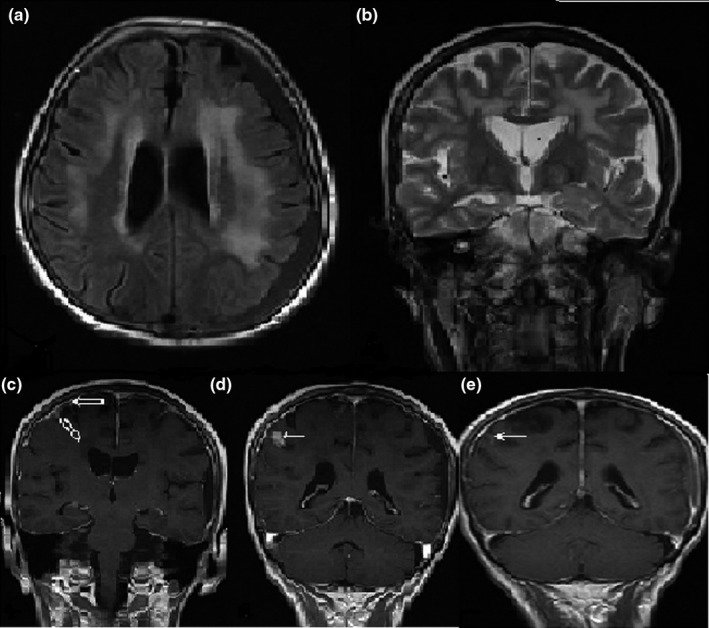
Axial FLAIR (a) and coronal T2‐weighted (b) images of patient with sensorineural hearing loss showed diffuse hyperintense signal changes bilaterally in the subcortical and periventricular deep white matter and the subdural hygroma on the left. In postcontrast coronal (c) series, diffuse dural and leptomeningeal (open arrow) enhancement and right frontal granuloma formation (white arrow) (d) were observed; After treatment, the granuloma size decreased (e) but leptomeningeal enhancement continued
